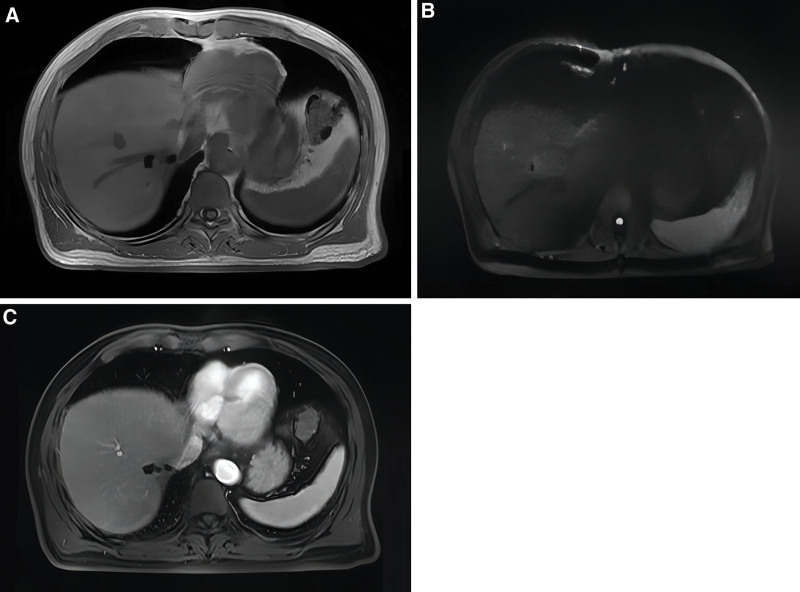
Postoperative MR image of the abdomen. (A) Numerous areas with patchy prolonged T1 signal shadows are observed in the surgical site. (B) A patchy high DWI signal is detected at the periphery of the surgical site in the left liver lobe. (C) The enhanced scan reveals nonsignificant enhancement.
